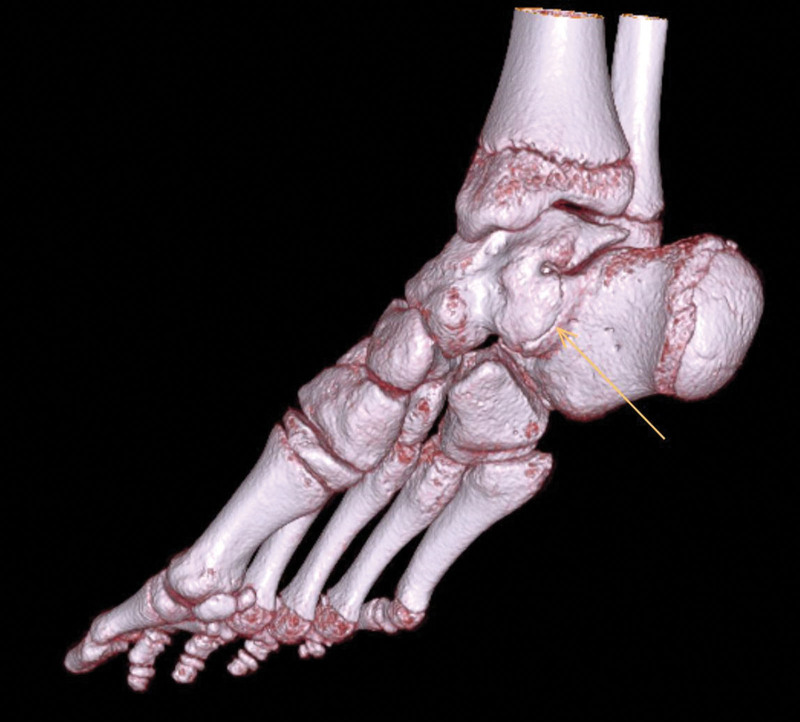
3D CT image of a talocalcaneal coalition.
